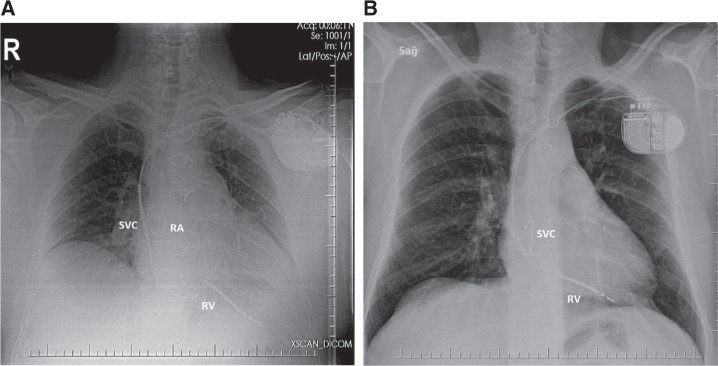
A: Postimplantation chest X-ray showing localization of the leads. B: In-office chest X-ray showing the SVC coil looped in the RA. SVC: superior vena cava; RA: right atrium; RV: right ventricle.
